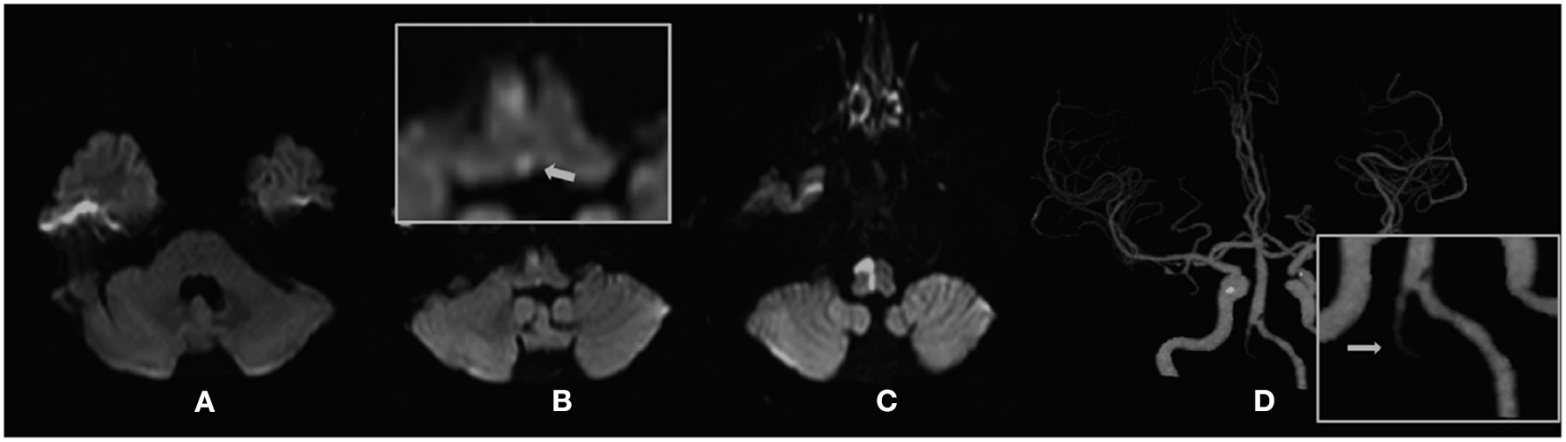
(A–C) Diffusion-weighted magnetic resonance imaging (DWI) of the patient. (A) No abnormal signal is observed in the vestibular nucleus of the pons. (B) Acute infarction in the medial medulla, which involves the nucleus prepositus hypoglossal (NPH) (white arrow). (D) Cranial computed tomography angiography (CTA) of the patient demonstrates total occlusion of the left intracranial vertebral artery (ICVA), which represents the offending vessel.
